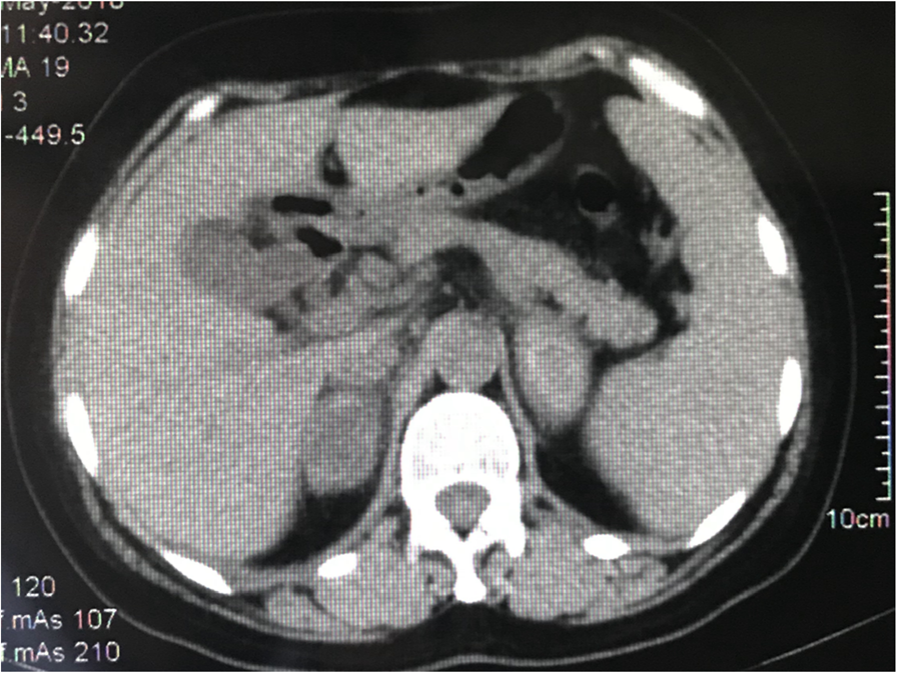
Axial computed tomography scan abdominal (postoperative day 12) revealed a bilateral adrenal mass with mixed high density
